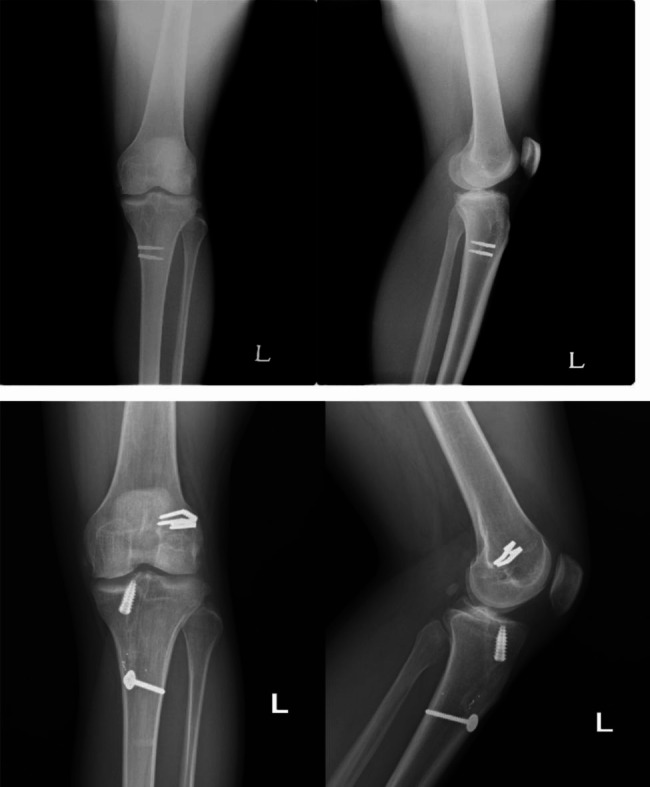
Preoperative and postoperative radiography of revision OA-ACLR
